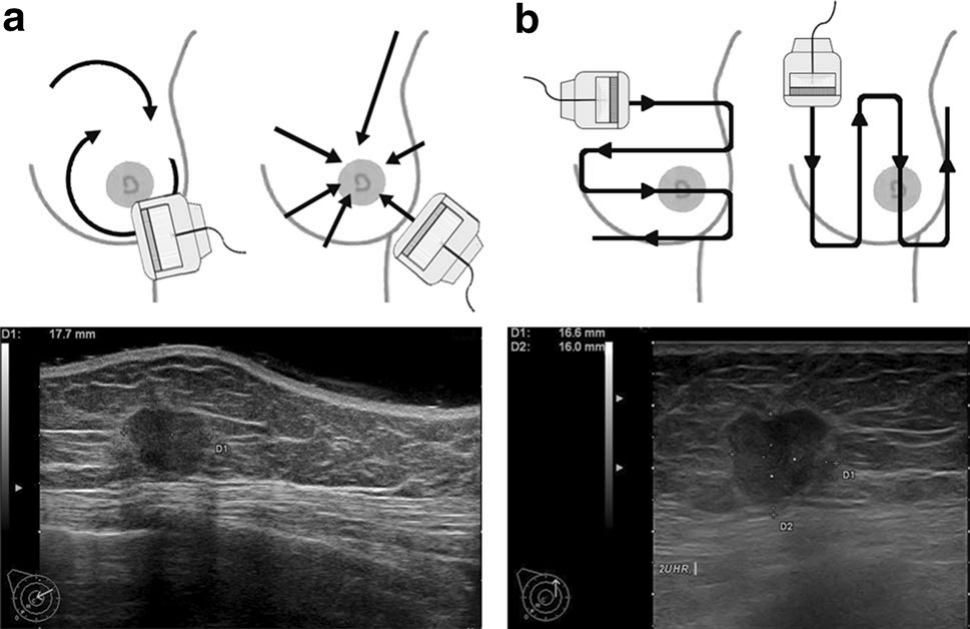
Radial and meander-like ultrasound. a Scheme of radial scanning movement (top) and corresponding ultrasound image (bottom). Left panel: radial movement and radial movement for the axillary tail; right panel: anti-radial movement. b Scheme of meander-like scanning movement in two orthogonal planes (top) and corresponding ultrasound image (bottom). Both ultrasound images are from the same 59-year-old patient diagnosed with invasive ductal breast cancer
